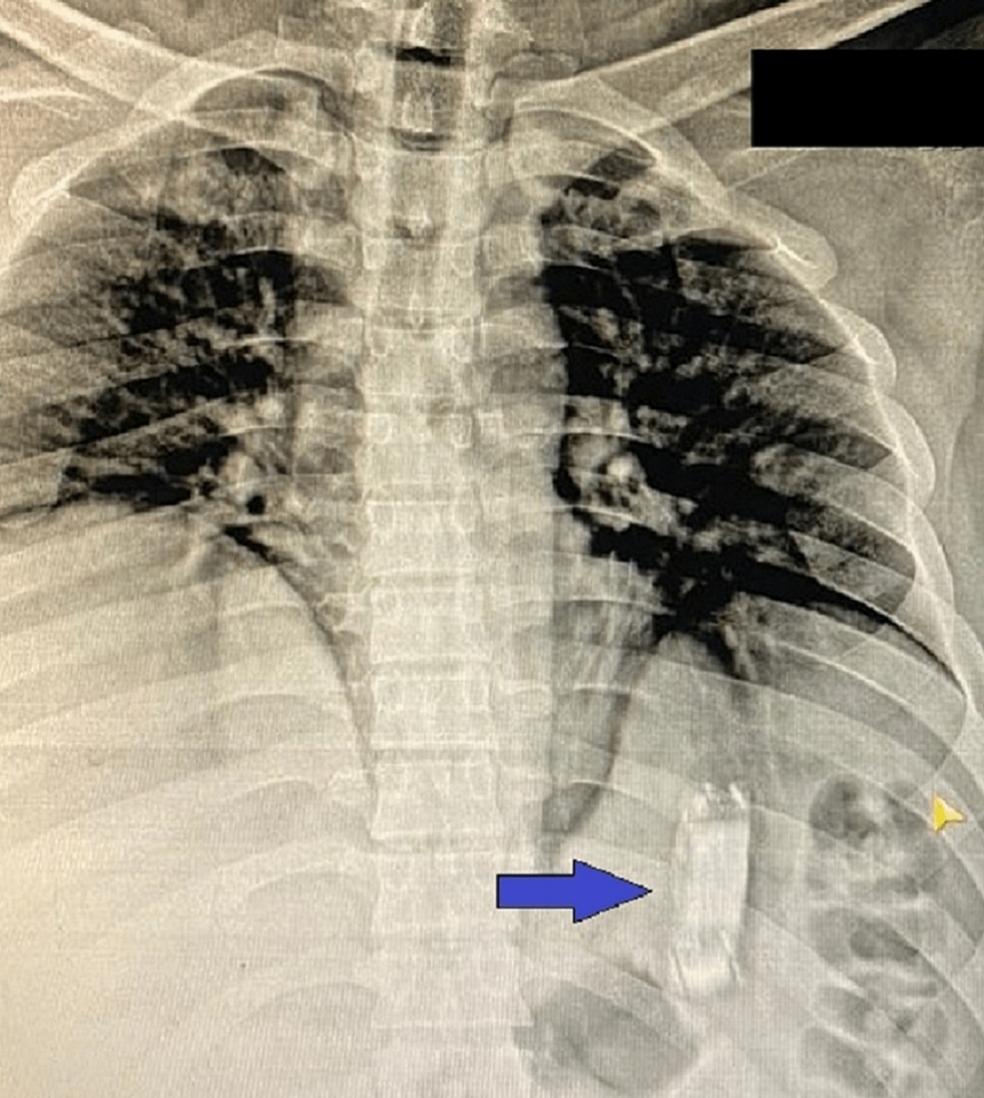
X-ray of chest and upper abdomen, with a blue arrow pointing to the mobile phone in the stomach
